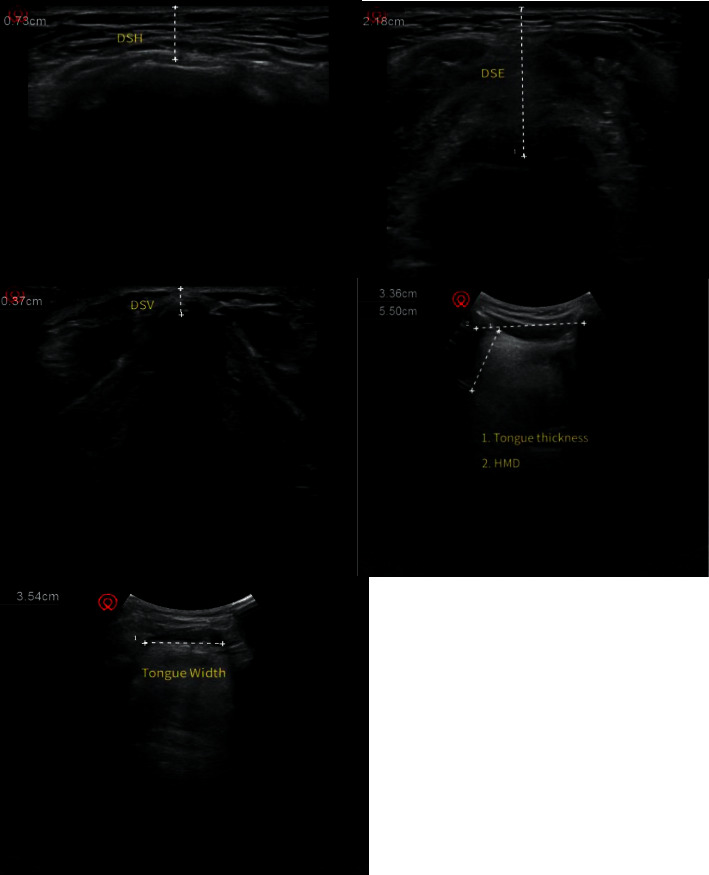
Ultrasonic images of each plane.
